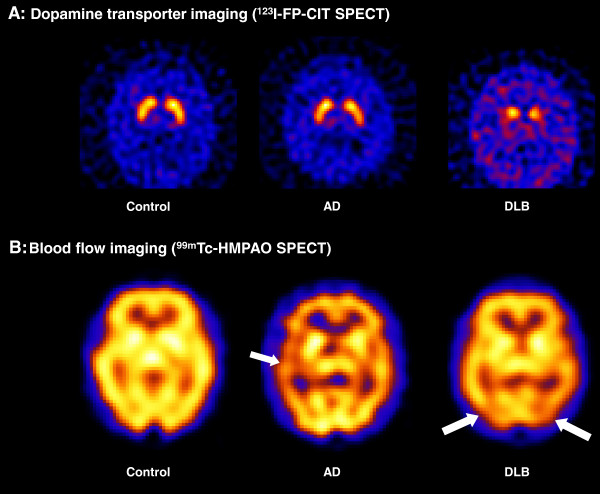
Examples of imaging abnormalities in dementia with Lewy bodies. (A) Dopamine transporter imaging. Both the control and Alzheimer’s disease (AD) subjects display normal dopamine transporter levels in the striatum. The dementia with Lewy bodies (DLB) subject displays reduced uptake in the putamen bilaterally, reflecting nigrostriatal degeneration. (B) Cerebral blood flow imaging. The AD subject shows decreased perfusion compared with control, particularly in the left temporal lobe. The DLB subject also exhibits perfusion deficits, but these are largely confined to posterior regions including the occipital lobes. FP-CIT, *N*-ω-fluoropropyl-2β-carbomethoxy-3β-(4-iodophenyl)nortropane; HMPAO, exametazime; SPECT, single photon emission computed tomography.
